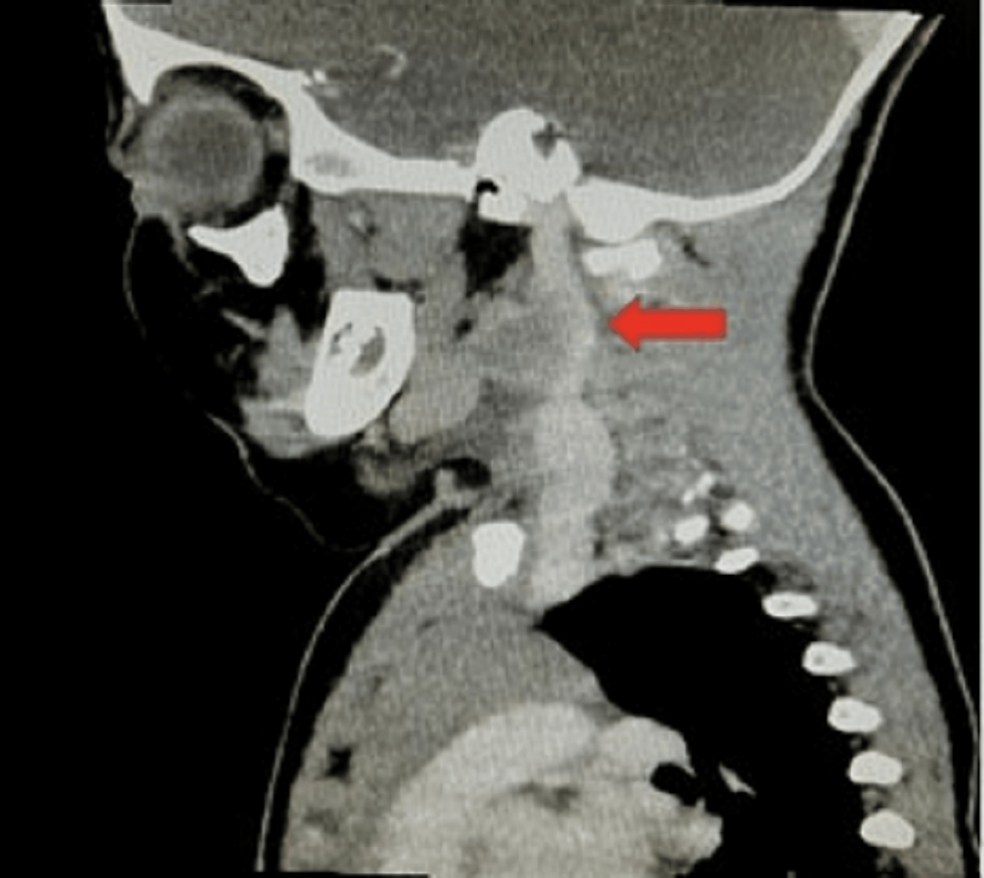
CT with contrast sagittal section showing heterogeneous attenuation on the left anterior cervical area of the neck (pointed by red arrow)
